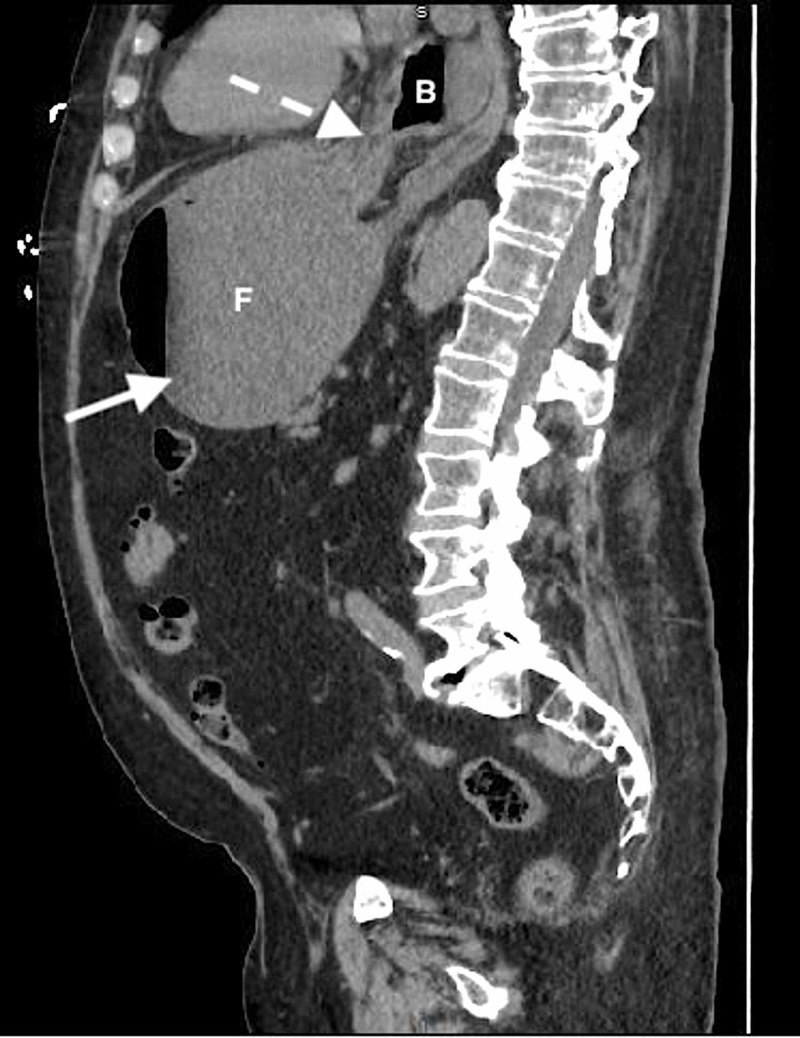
CT of the abdomen/pelvis (sagittal view) demonstrating gastric volvulus with a fluid-filled distended stomach (solid arrow) and malrotation (dotted arrow). B, gastric body; F, gastric fundus.
